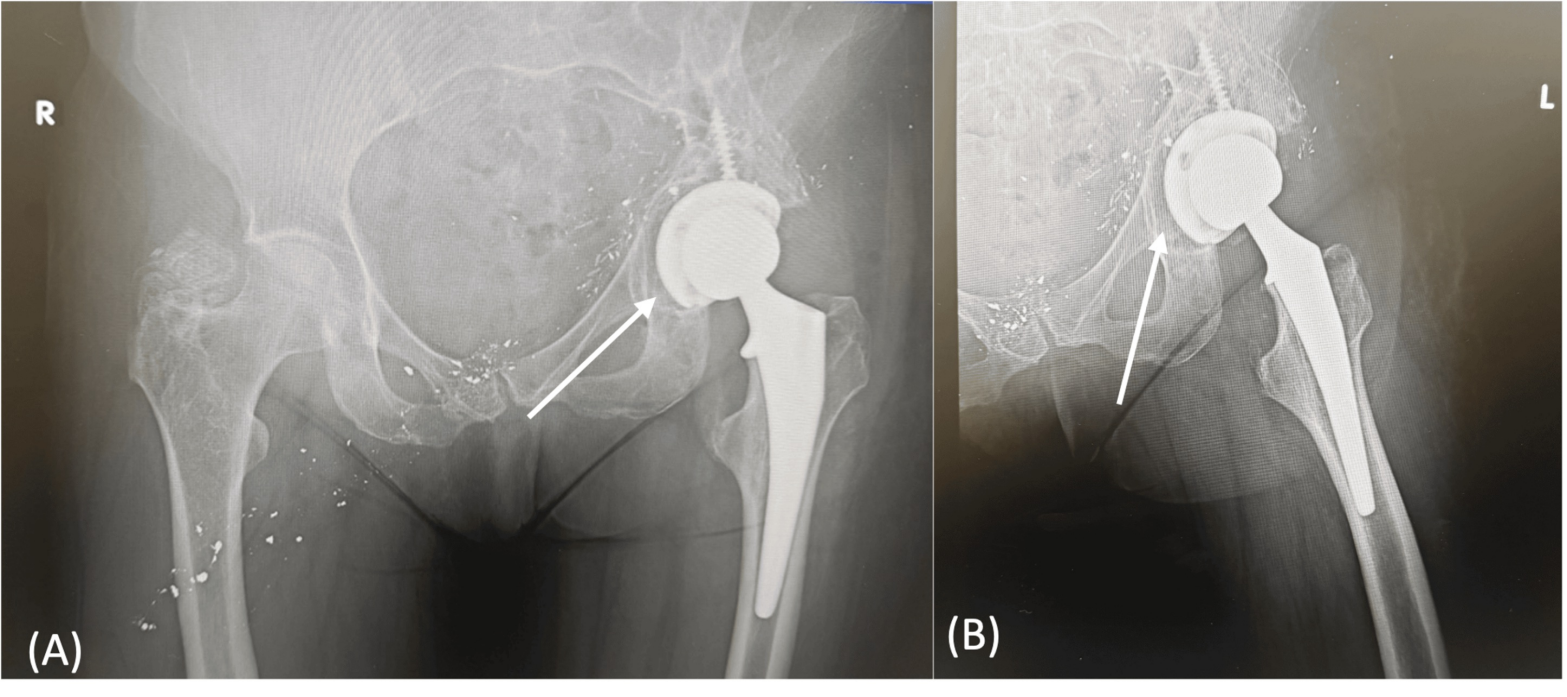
Postoperative hip radiographs. (A and B) Anteroposterior pelvis radiograph demonstrating stable positioning of left THA components without evidence of loosening or subsidence. THA: Total hip arthroplasty
